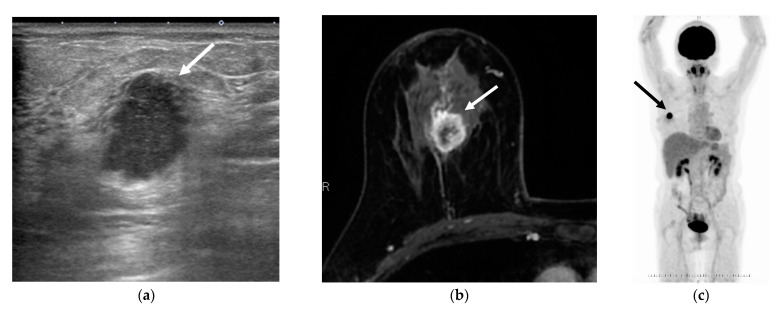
A woman in her 60s was found to have a right invasive ductal carcinoma of no special type by surgery (arrows). (a) Breast ultrasonography (B mode), (b) contrast-enhanced breast magnetic resonance image (axial image of early phase of contrast-enhanced), and (c) whole-body positron emission tomography. Pathological analysis revealed T1cN0M0, nuclear grade 3, negative estrogen receptor, negative progesterone receptor, and negative human epidermal growth factor receptor 2 (not shown). She was found to have an SUVmax of 12.5, anatomical stage IA, clinical prognostic stage IB, and pathological prognostic stage IB.
